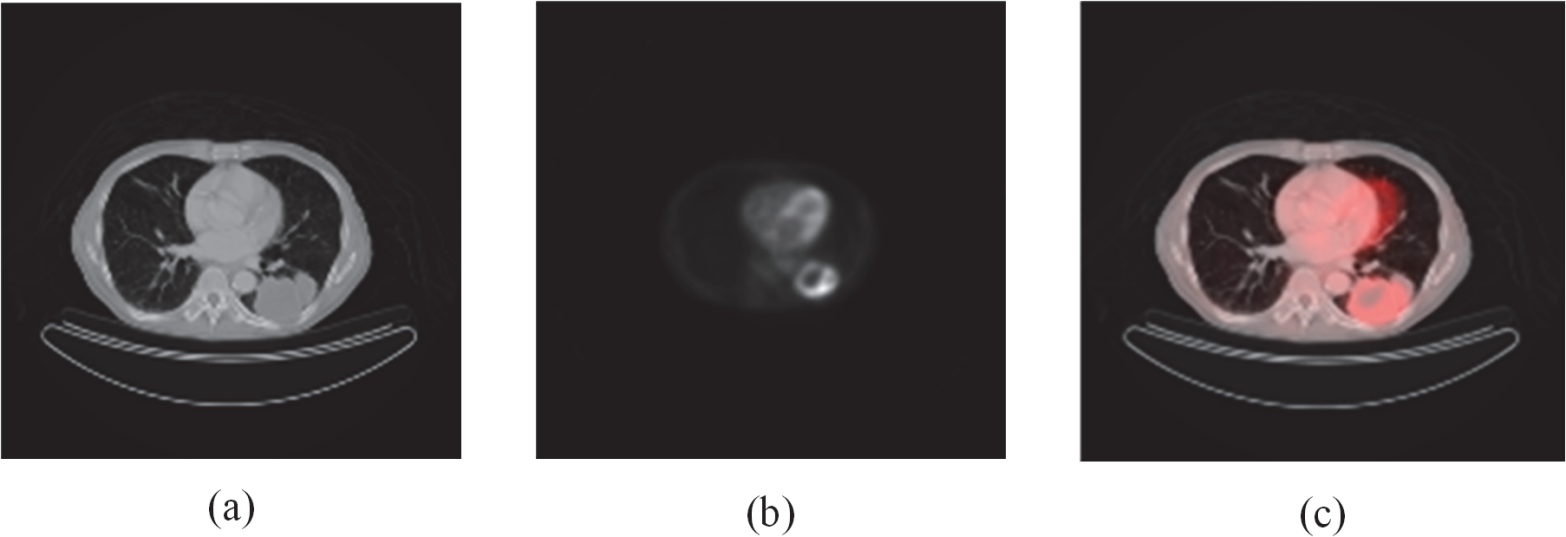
Results of PET/CT image registration. (a) CT image; (b) PET image at the same position; (c) results of image registration.
